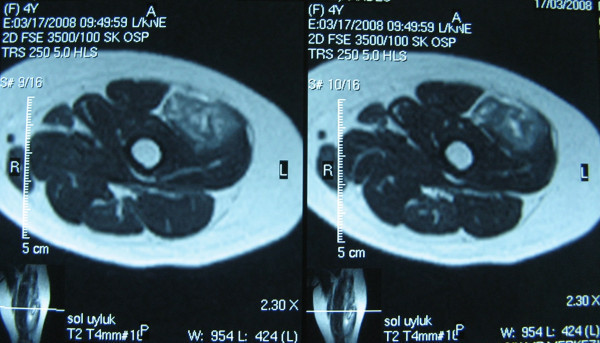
Axial MR images show tear in vastus lateralis muscle.
